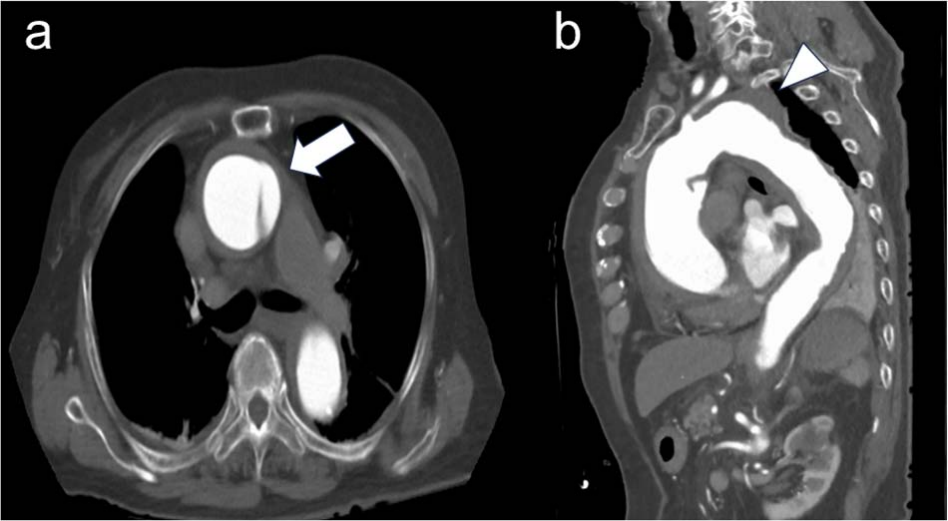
Preoperative computed tomography imaging. (a) There was a flap at the ascending aorta, a finding of acute aortic dissection of Stanford type A (white arrow). (b) A 54 mm large thoracic aortic aneurysm was found at the distal arch aorta (white arrow head).
